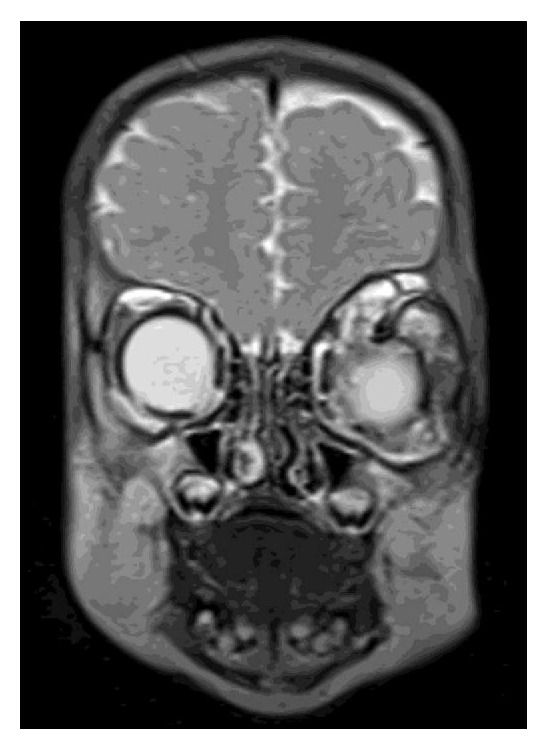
Orbital CT scan at one year of age showing the proptosis of the globe and some cystic spaces between the extraocular muscles in the left orbit.
